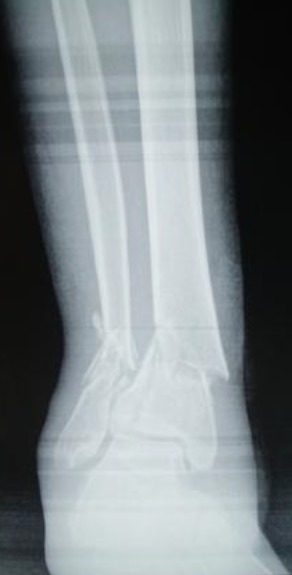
Radiographie de la jambe droite de face montrant la fracture du pilon tibial
